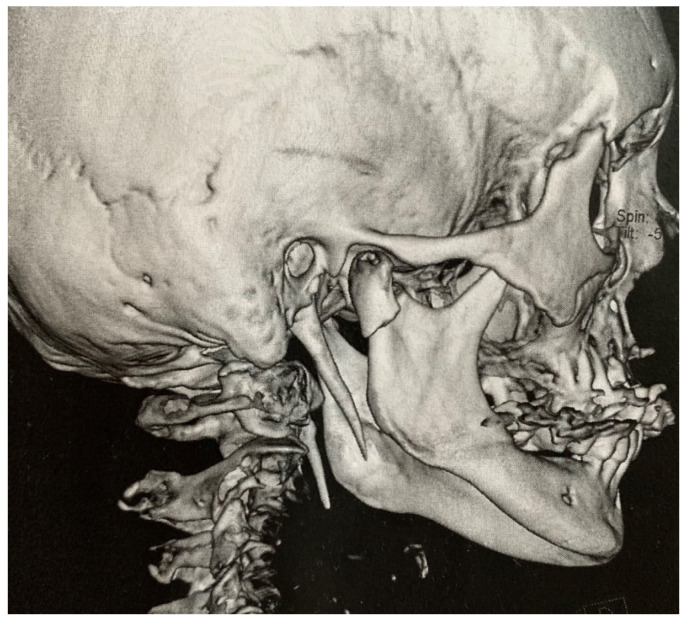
Three-dimensional computed tomography —an accidental finding of elongated styloid process in an asymptomatic patient with a fracture of the articular process of the mandible.
